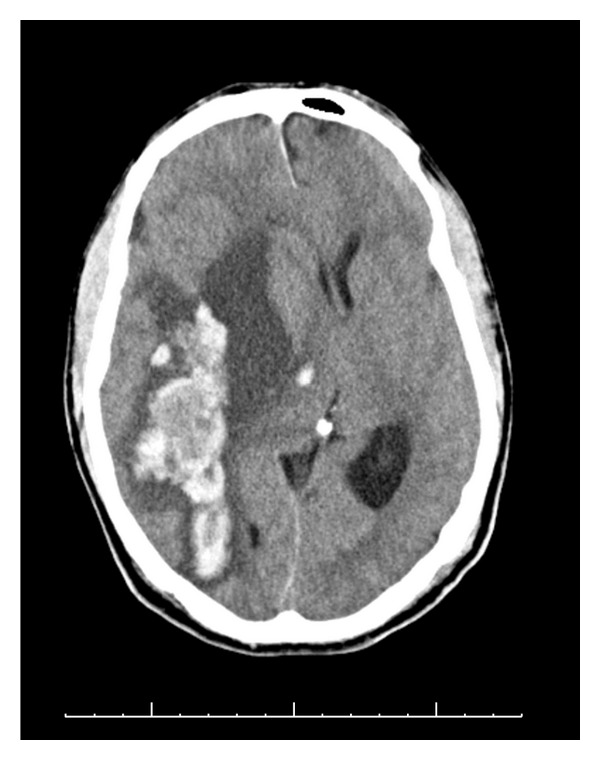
CT of the head, performed within 30 minutes of presentation, reveals a large intraparenchymal hemorrhage in the right cerebral hemisphere involving the right parietotemporal lobes. There is transfalcine herniation and a right to left midline shift measuring 1.9 cm.
